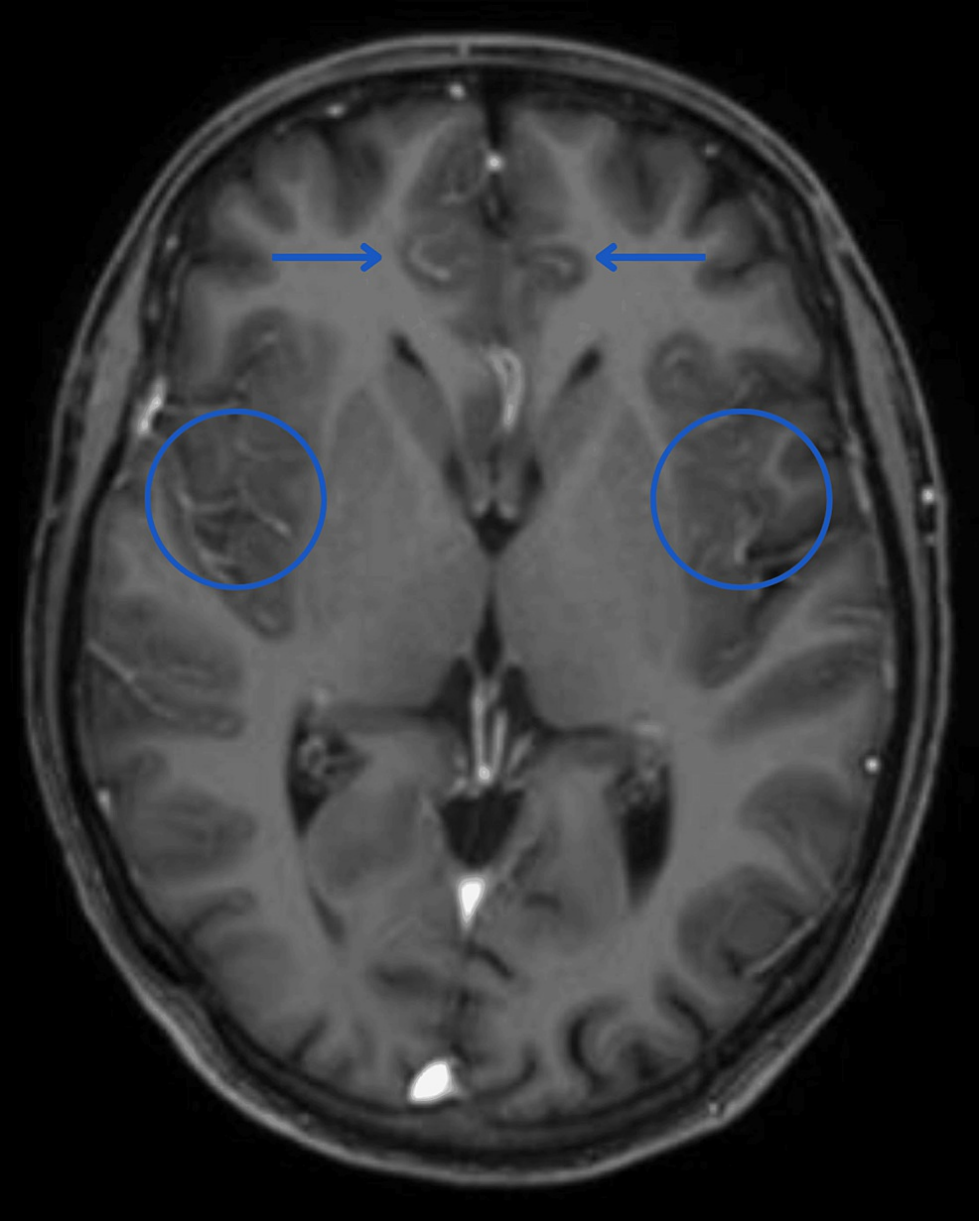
MRI-Brain revealing acute hyperammonemic or acute hepatic encephalopathy MRI: Magnetic Resonance Imaging Blue arrows point to the non-enhancing symmetrical signal abnormalities with diffusion restriction in the bilateral parasagittal frontal region involving the cingulate gyrus. Blue circles reveal non-enhancing symmetrical signal abnormalities with diffusion restriction in the bilateral parasagittal frontal region involving the bilateral insular cortex.
